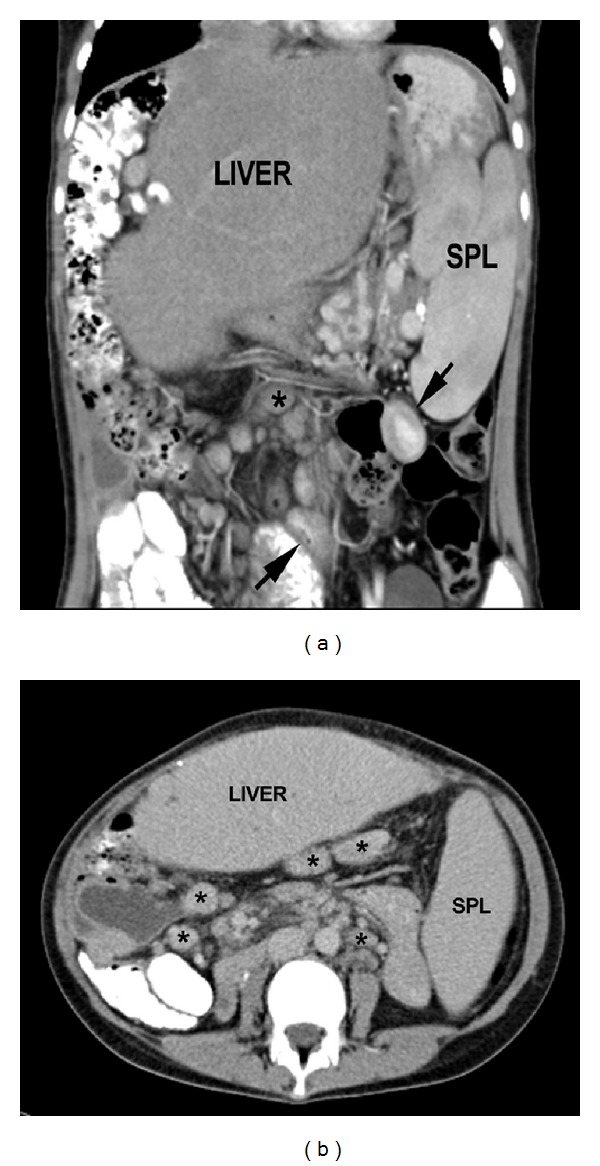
Axial (a) and coronal reformats of contrast-enhanced abdominal CT showing marked enlargement of the spleen and transplanted liver, multiple moderately enlarged mesenteric and gastrohepatic ligament lymph nodes (∗), and focal small bowel wall thickening (arrows).
